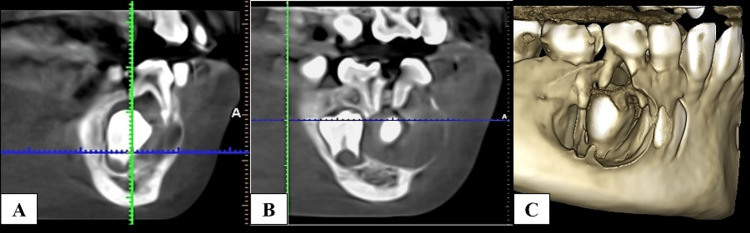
CBCT images: (A) an expansile unilocular lesion involving the posterior part of the body of the mandible; (B) the bilateral cortical plate expansion and the second primary molar (45) was unusually pushed into an ectopic position within the radiolucency distally; (C) the involvement of mesial root of the second primary molar (85) within the lesion. CBCT, cone-beam CT
